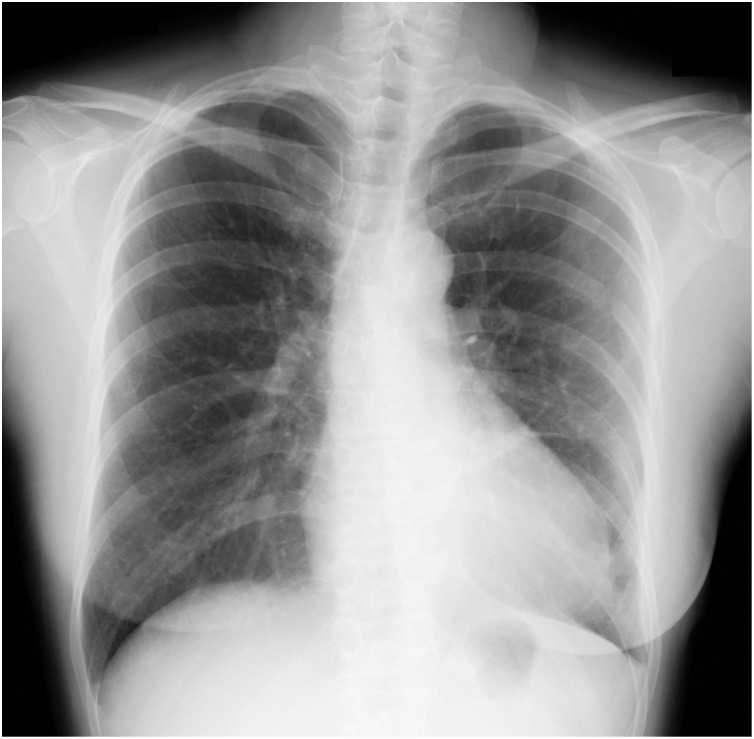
Chest radiograph at 3 months postoperatively demonstrating full lung re-expansion.
